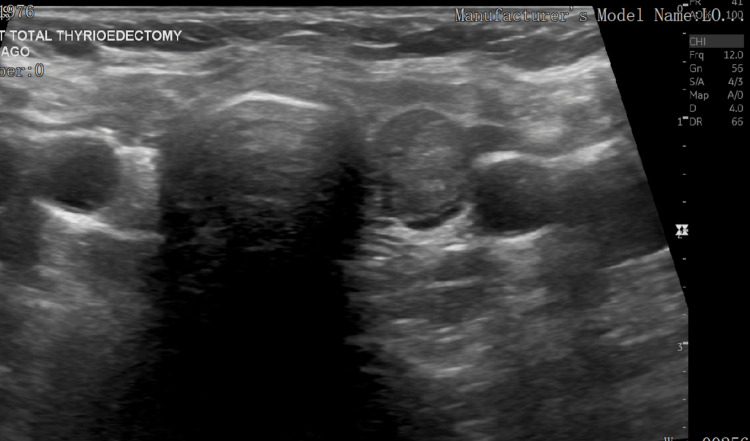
A neck ultrasound scan showing a midline exophytic thyroid nodule measuring about 2.8 x 16 cm
